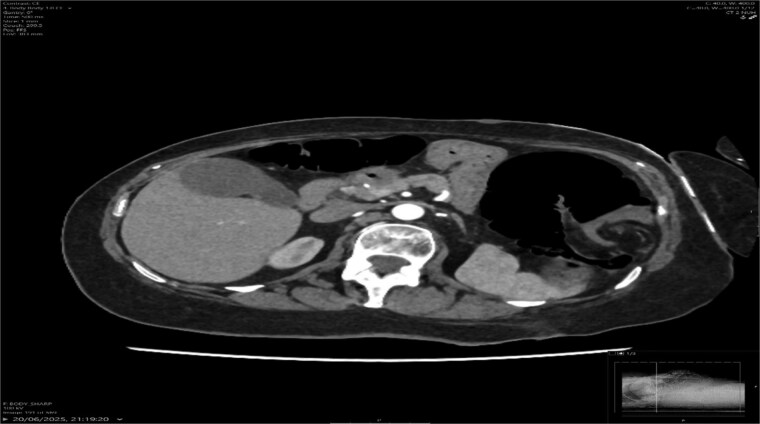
Axial CT showing the swirl sign.
